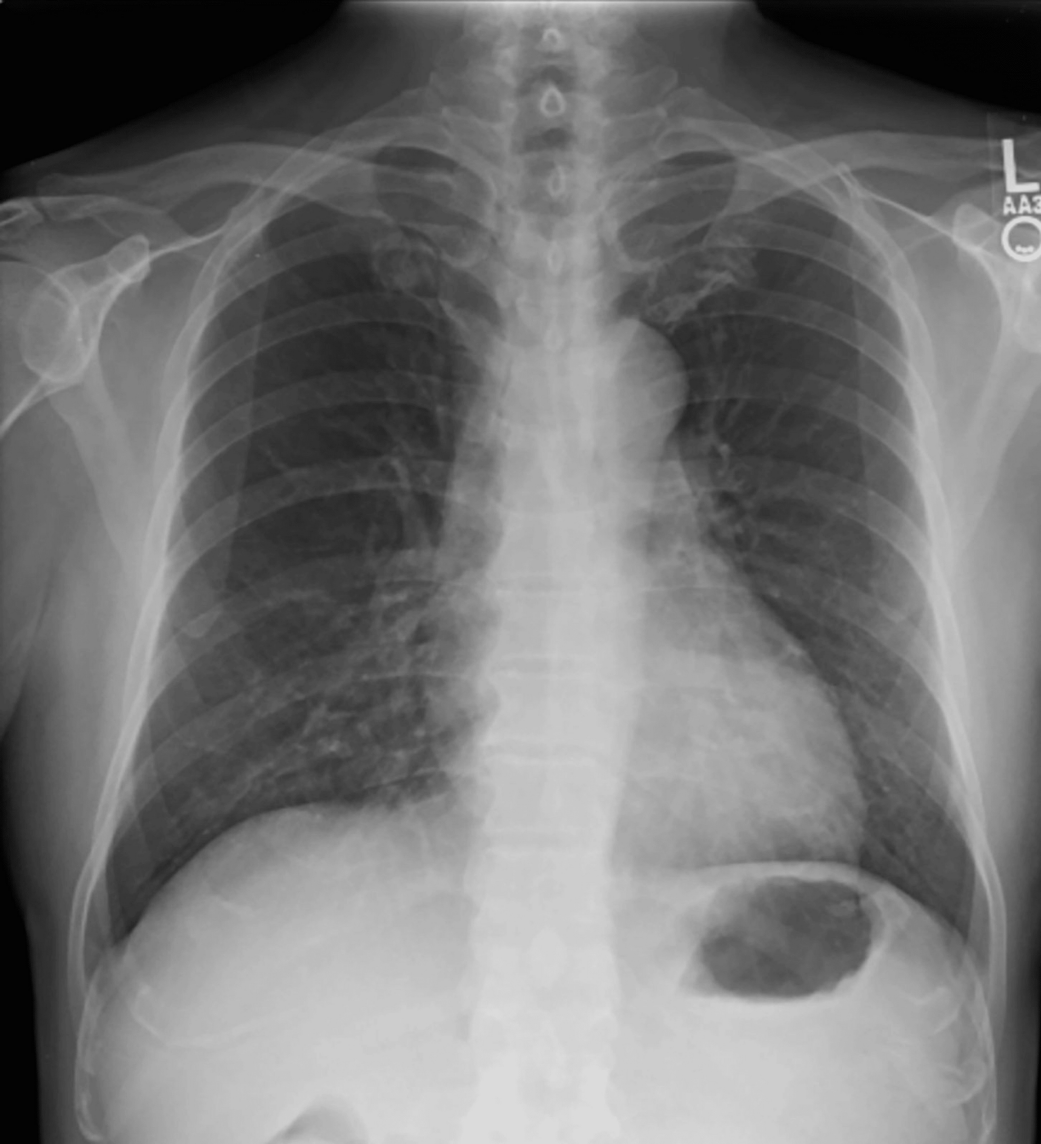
Chest radiograph Chest radiography demonstrating bilateral perihilar interstitial infiltrates
